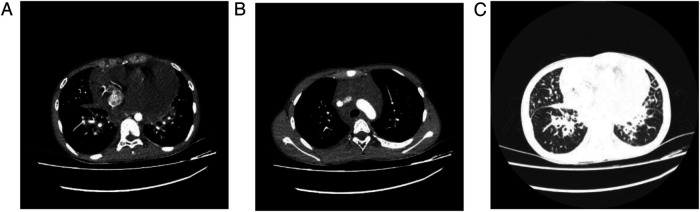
(A) Demonstrates extensive pleural effusion and pericardial effusion on the patient's pulmonary CT scan; (B) reveals interstitial thickening; and (C) shows diffuse ground-glass opacities within the lungs.
